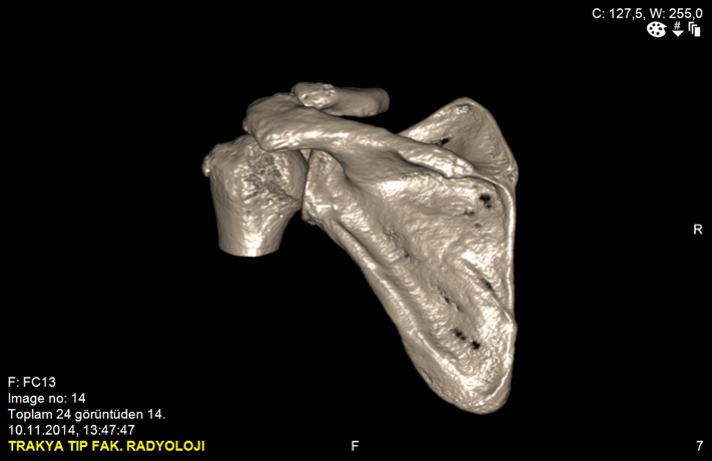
Follow-up computed tomography (three dimensional)
